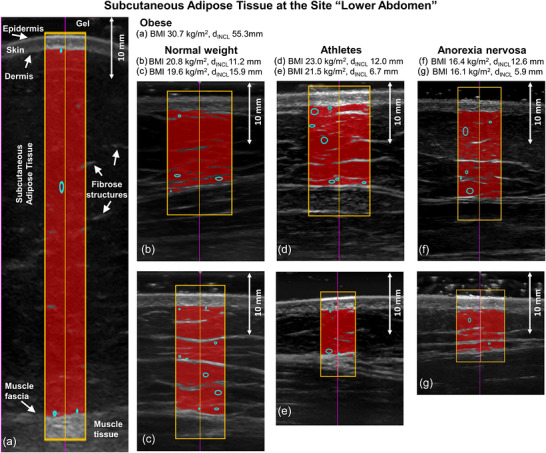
Differences in subcutaneous adipose tissue (SAT) thicknesses of the study cohort. The figure shows a comparison of SAT layers derived by the direct and highly accurate ultrasound method in different participants at the measurement site lower abdomen. The black area at the top of the ultrasound image represents a thick ultrasound gel layer applied to prevent tissue compression. The skin (epidermis and dermis) is clearly visible (two bright lines on top of the image), followed by the SAT layer, including diverse fibrous structures (fasciae) in the tissue (bright lines within this layer). SAT is highlighted in red; the lower border is the muscle fascia. (a) Obese participant: BMI 30.7 kg/m^2^; *d*
